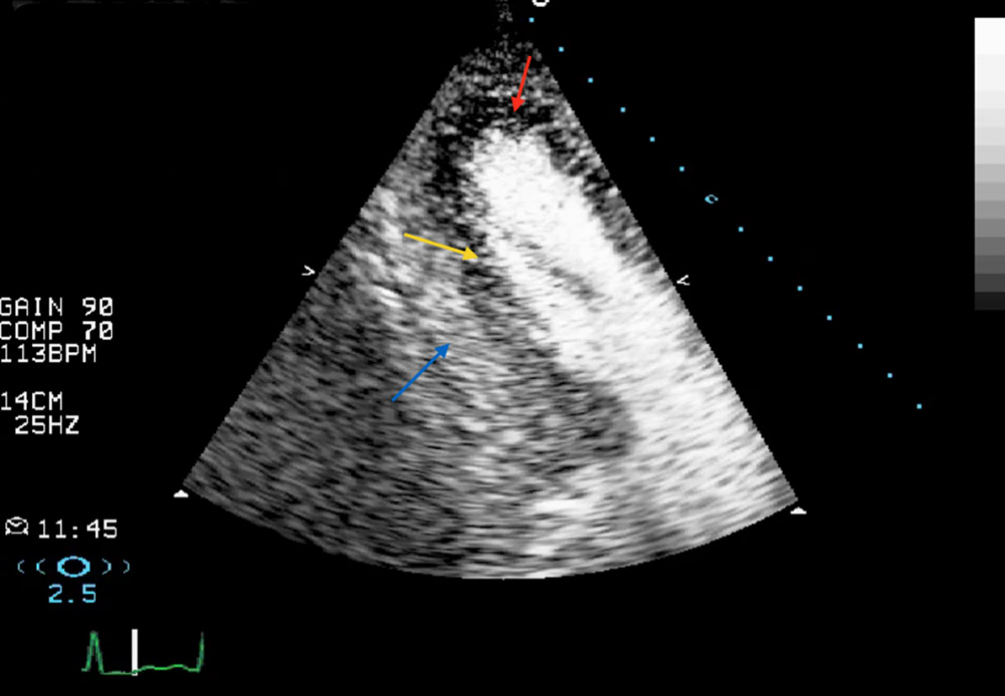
Another case of real-time low-MI myocardial contrast echocardiography that demonstrates a myocardial perfusion defect in an apical two-chamber view. *Blue arrow* epicardial wall, *yellow arrow* subendocardial perfusion defect, *red arrow* transmural perfusion defect. Coronary angiogram showed significant right and left coronary artery disease
